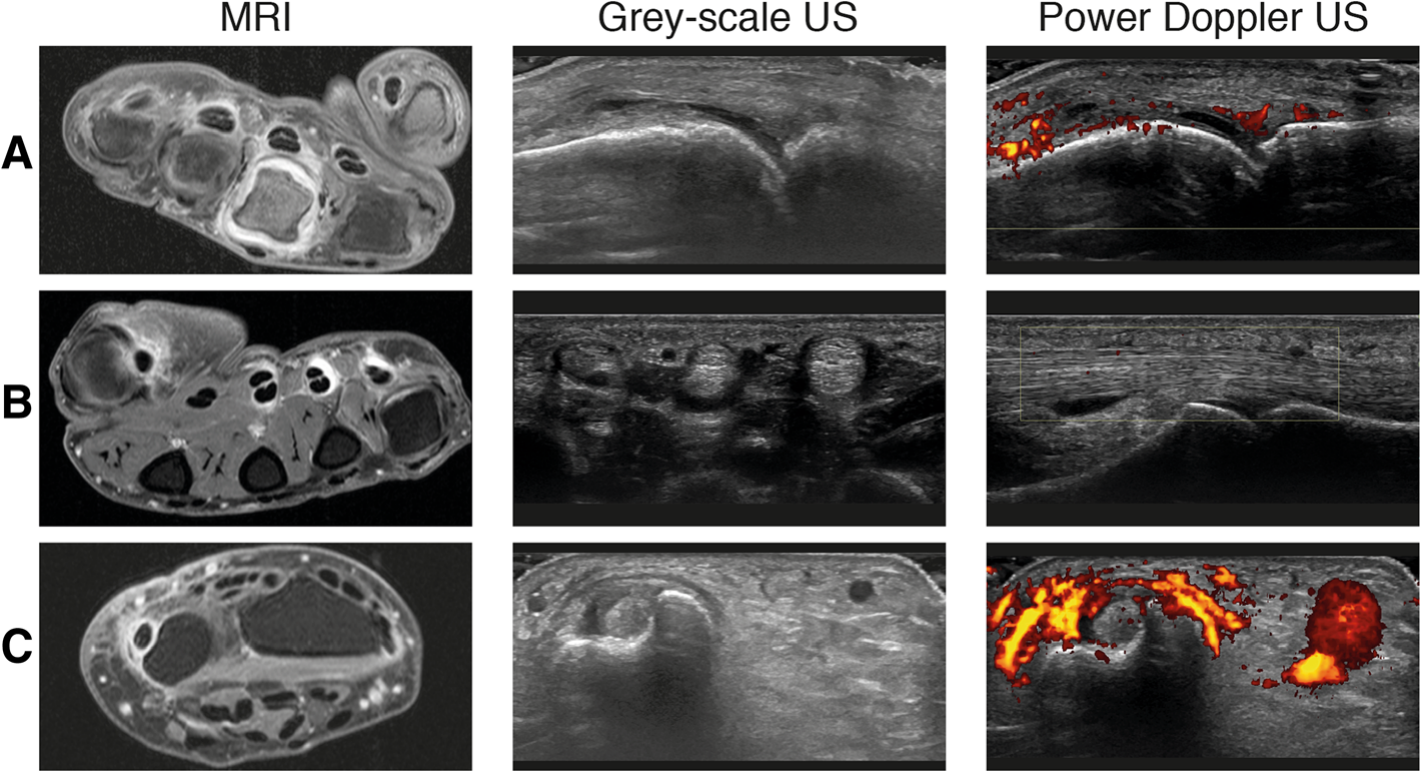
Examples of MRI-detected synovitis and tenosynovitis with corresponding greyscale and power Doppler ultrasound images. Examples of inflammation identified by MRI that were verified through ultrasound (US). Even though sometimes more inflammation was present on the MRI scan, we choose to show the corresponding US images of only one joint or tendon that was present on the image. a An example of synovitis MCP-3 by MRI, which was confirmed by greyscale (GS) and power Doppler (PD) US images of the same joint. b Tenosynovitis of the flexor of MCP-4 which was confirmed by GS but not by PD on US. c Inflammation of the extensor carpi ulnaris tendon at the wrist level which was confirmed by both GS and PD on US
